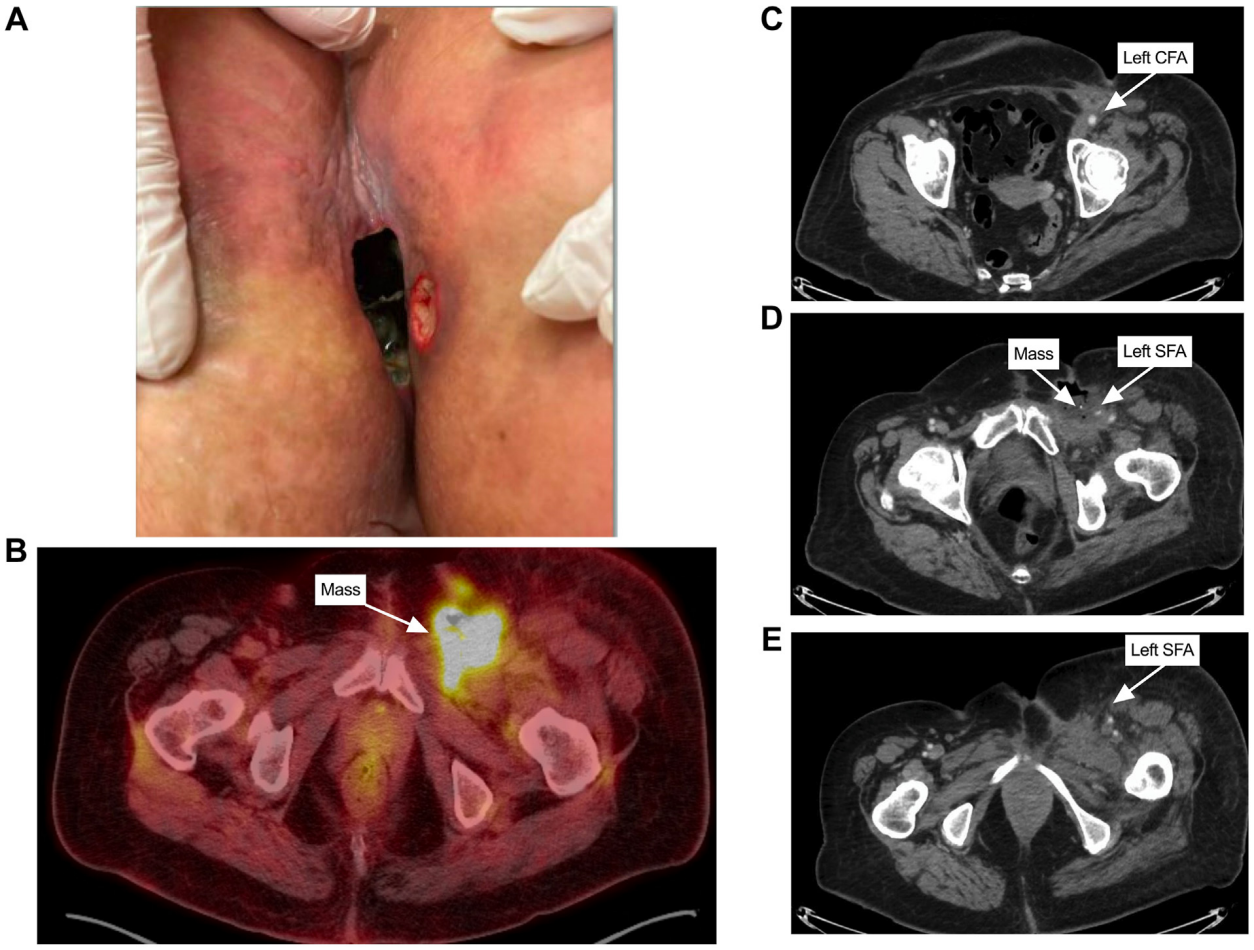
Preoperative wound and imaging. (A) Left groin infected and necrotic wound at presentation. (B) Preoperative positron emission tomography scan at the level of the left groin mass. (C) Axial cross-sections from the preoperative computed tomography (CT) angiography at the level of the (C) common femoral artery (*CFA*), (D) left groin necrotic mass, (E) superficial femoral artery (*SFA*) below the level of the mass showing compression of the left SFA.
